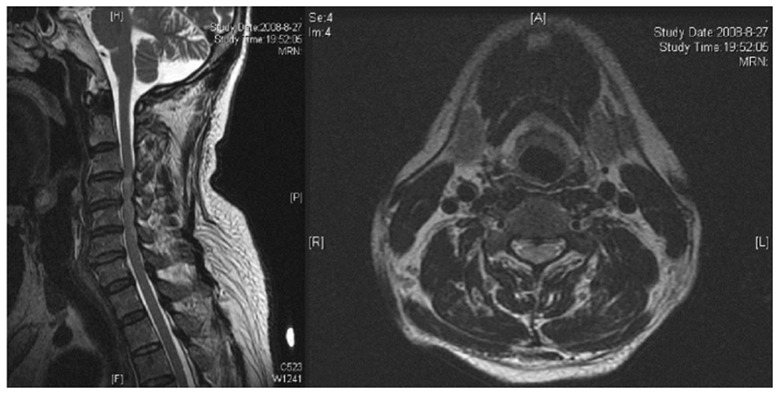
Sixty-one-year-old male exhibiting high signal spinal cord changes at the C3–4 level with T2-weighted magnetic resonance imaging (MRI).
